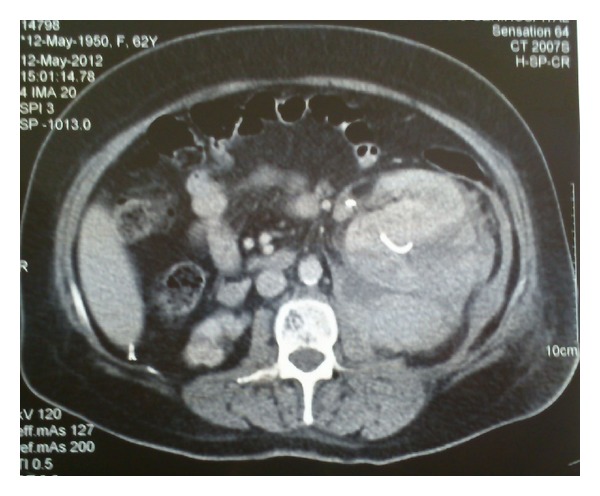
CT of the abdomen with i.v. contrast showing a 12 × 8 perirenal hematoma pushing anteriorly the left kidney with the tip of the stent penetrating the renal parenchyma.
